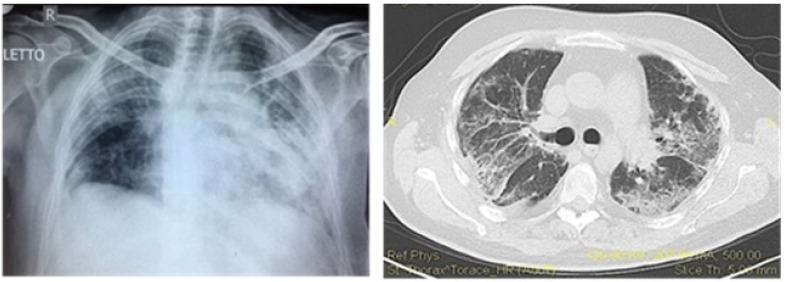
Diffuse alveolar hemorrhage. A chest radiography shows bilateral widespread infiltrates in both medium lower fields. A chest computed tomography shows left pulmonary embolism and massive diffuse infiltration of both lung fields. R means right.
